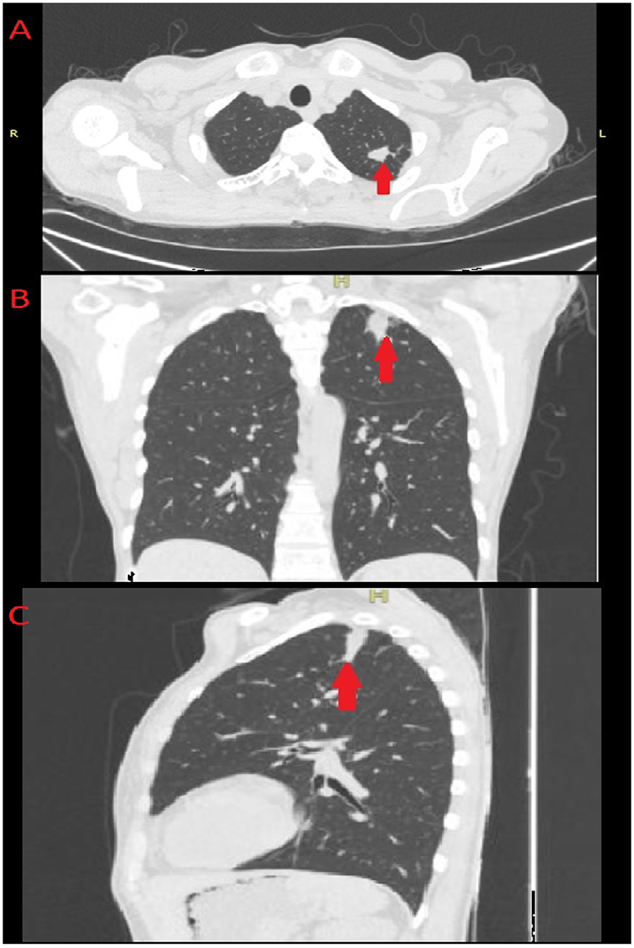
CT of chest - Non-enhancing lobulated mass located in the left upper zone posterolateral without cavitation suggestive of a differential of infective pulmonary granulomatous disease. A – Axial slice. B – Coronal view. C – Sagittal view.
